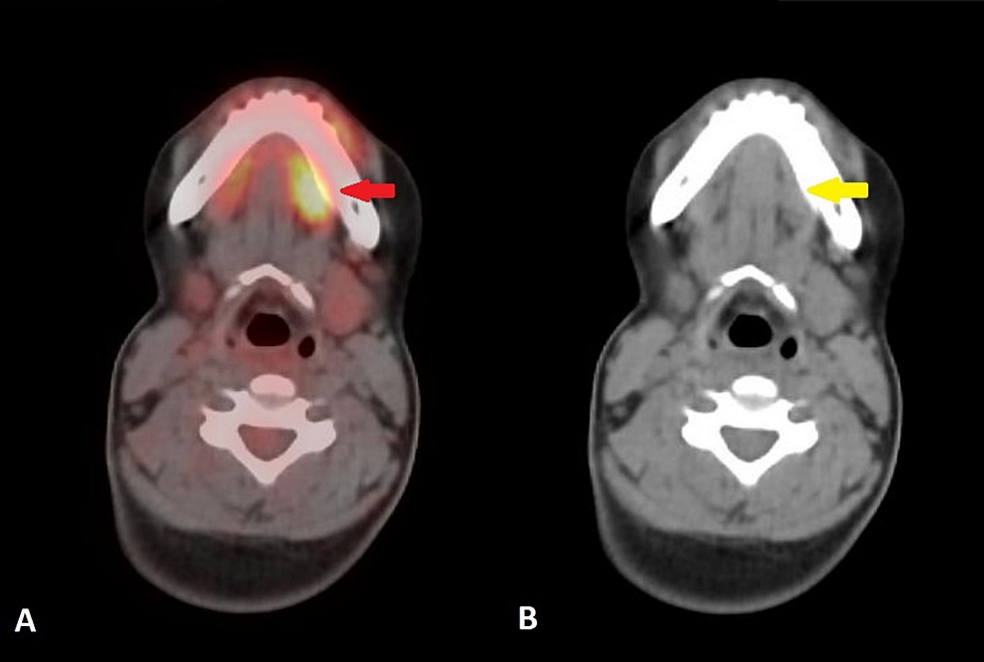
18F-FDG PET/CT axial images of head and neck region (A) fused image (B) CT image (A) Fused image shows linearly orientated 18F-FDG uptake in the floor of the mouth, projecting over the left mylohyoid (red arrow) (B) CT image shows normal appearance of the left mylohyoid muscle (yellow arrow) without any soft tissue mass or enlargement. These findings represent physiologic uptake. 18F-FDG PET/CT: Fluorine-18-Fluorodeoxyglucose Positron Emission Tomography/Computed Tomography
